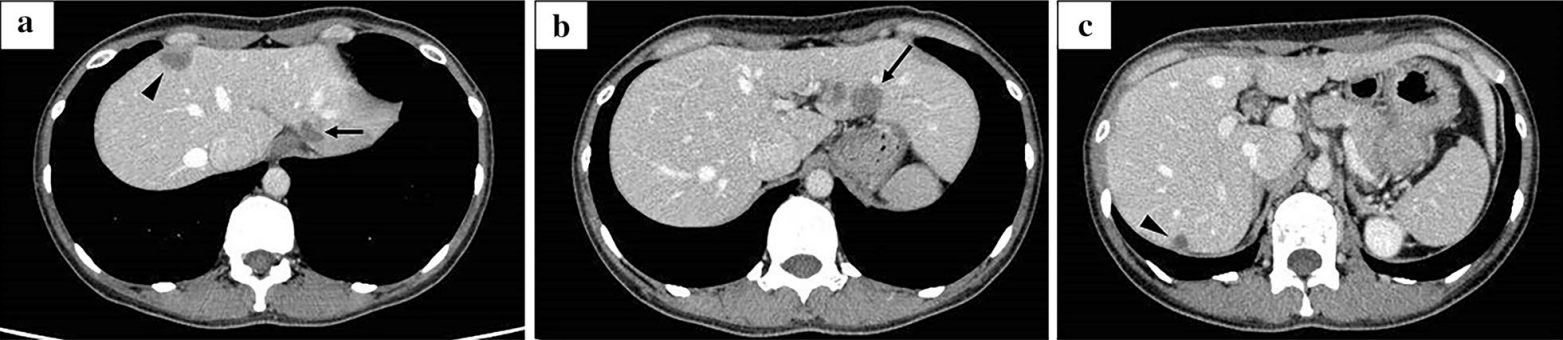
a–c Abdominal contrast-enhanced computed tomography showed four hypovascular liver tumors in the left lateral section (a and b, arrow), the posterior superior segment (S7) (c, arrowhead), and the anterior superior segment (S8) (Fig. 1a, arrowhead)
